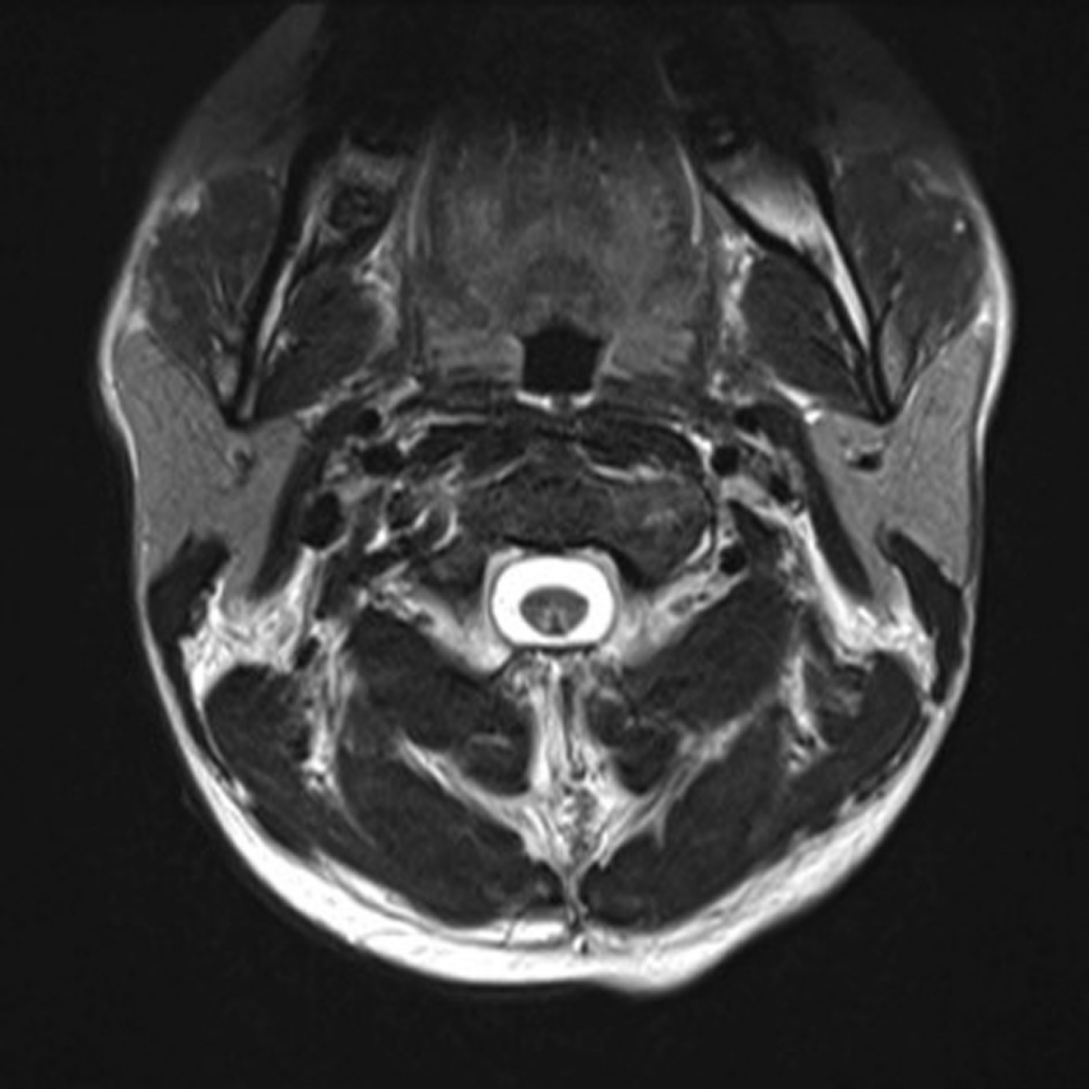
MRI axial T2‐weighted sequences of the cervical region, showing increased signal on the axial T2‐weighted sequence in the posterior columns at C2 and C3.
